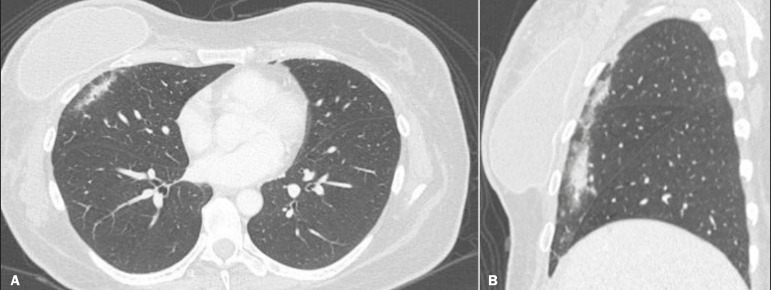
Axial and sagittal CT slices (A and B, respectively) showing peripheral ground-glass opacities in the region of the irradiated site in a patient who had undergone four weeks of radiotherapy of the right breast for the treatment of breast cancer.
